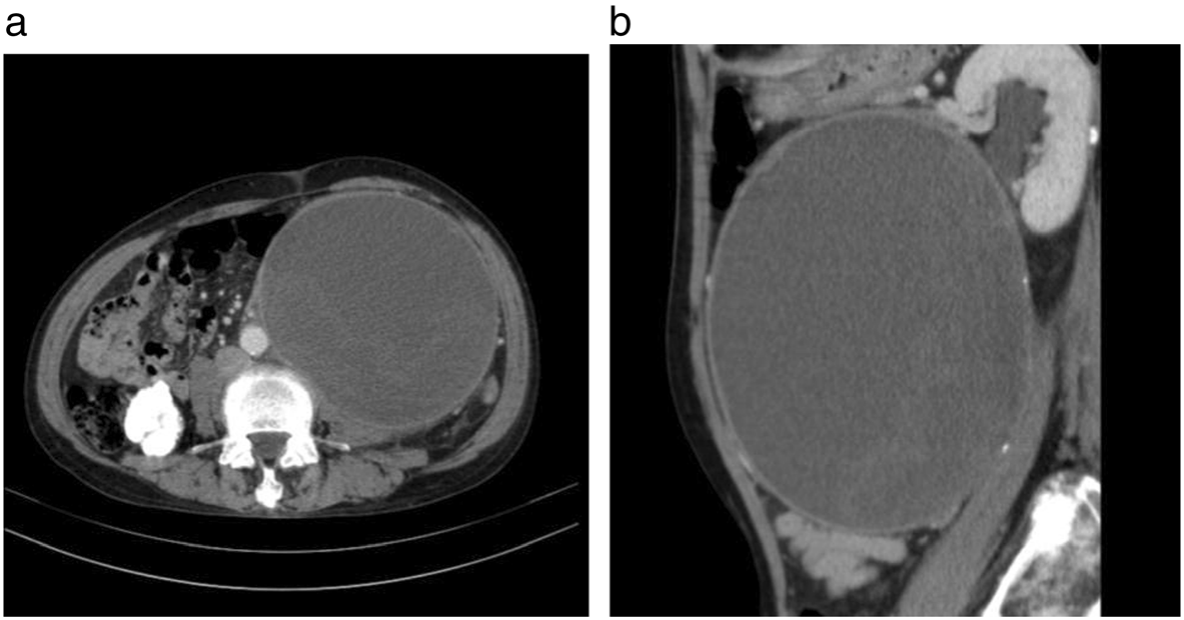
Enhanced abdominal computed tomography. (a) Axial view: The retroperitoneal mass is 20 cm × 15 cm × 13 cm in size and well-circumscribed. (b) Sagital view: The left kidney is compressed upward, and hydronephrosis is evident.
